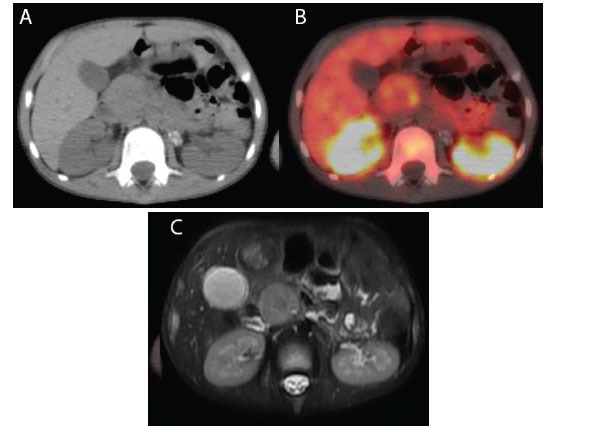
The uncinate process of the pancreas displays heterogeneous uptake and central relative low activity, as seen in the CT image (A) and corresponding Ga68-DOTATATE fusion image (B) of Case 1. Diffusion restriction and malignant involvement of the region are seen on the DW-MRI scan (C). In this instance, the pancreas was also involved in addition to the widespread illness. Following three months of imaging tests, he passed away.
